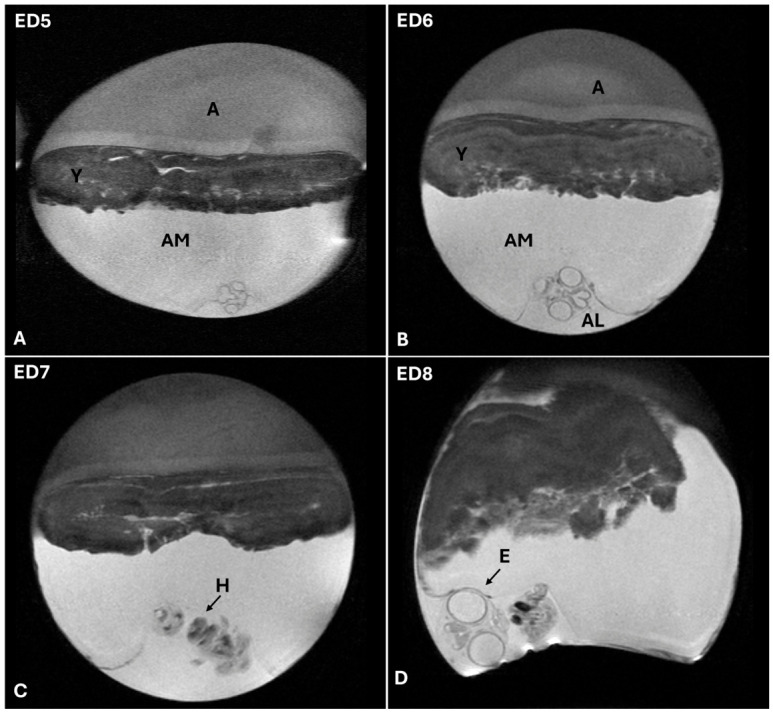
T2-weighted MRI images of CE between ED5 and ED8 showing clear differentiation of egg compartments (A,B) and progressive visualization of key embryonic structures, including heart (C) and eyes (D), in paracoronal planes. Abbreviations: AM, amniotic cavity; A, albumen; Y, yolk; AL, allantois; H, heart; E, eye.
